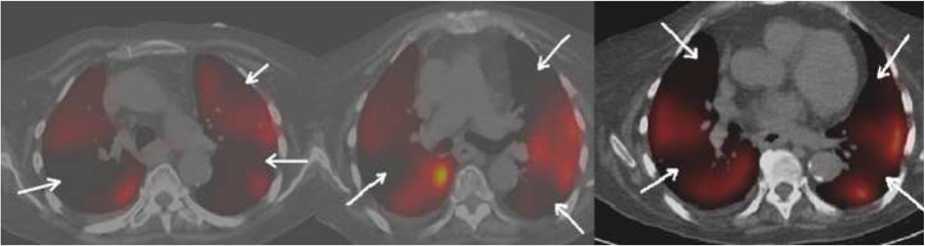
SPECT perfusion scan in a patient with CTEPH. SPECT demonstrates multiple perfusion defects (arrows)
